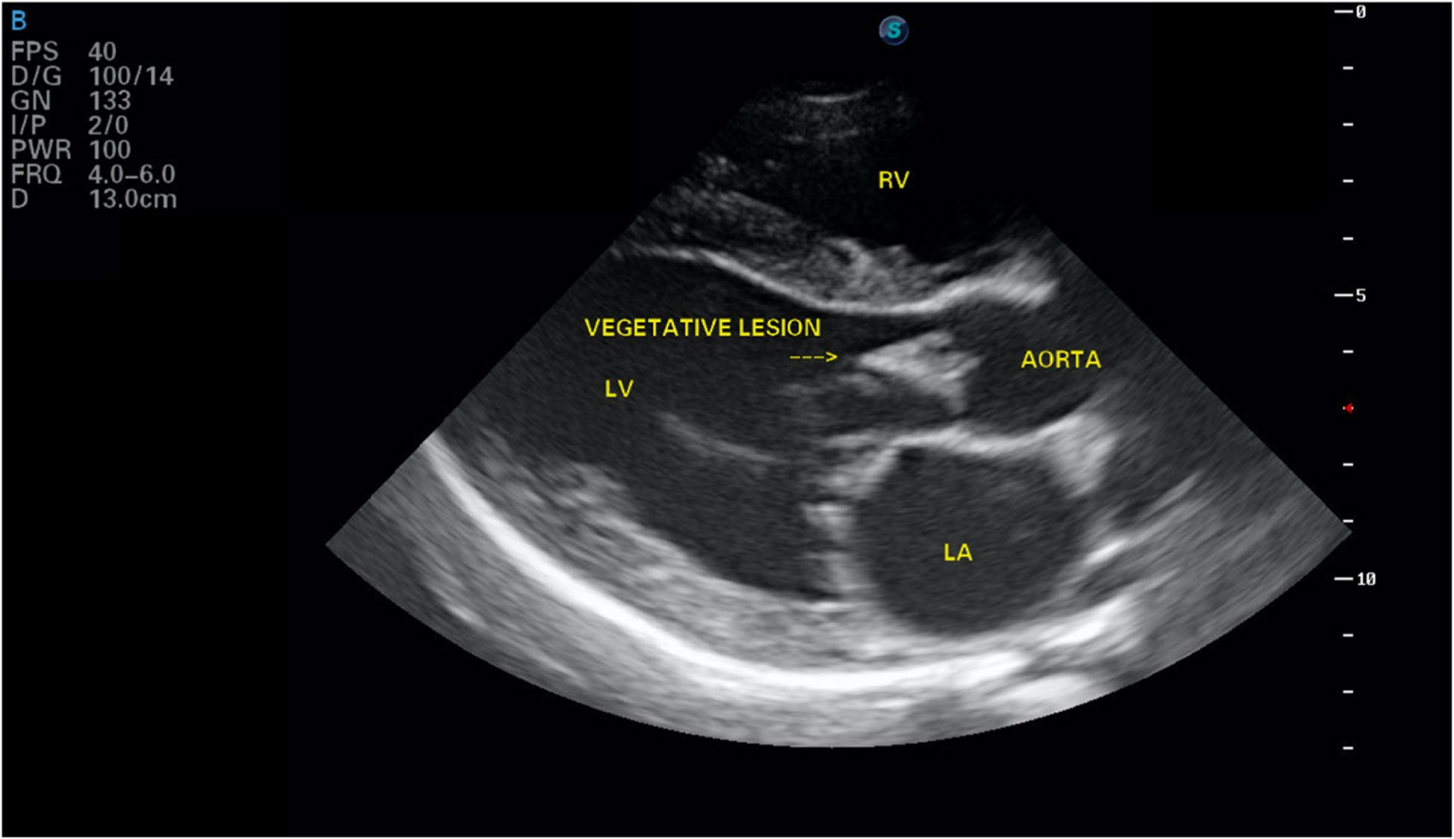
Aortic valve vegetation as seen on right parasternal long axis echocardiography (LA: left atrium, LV: left ventricle, RV: left ventricle)
